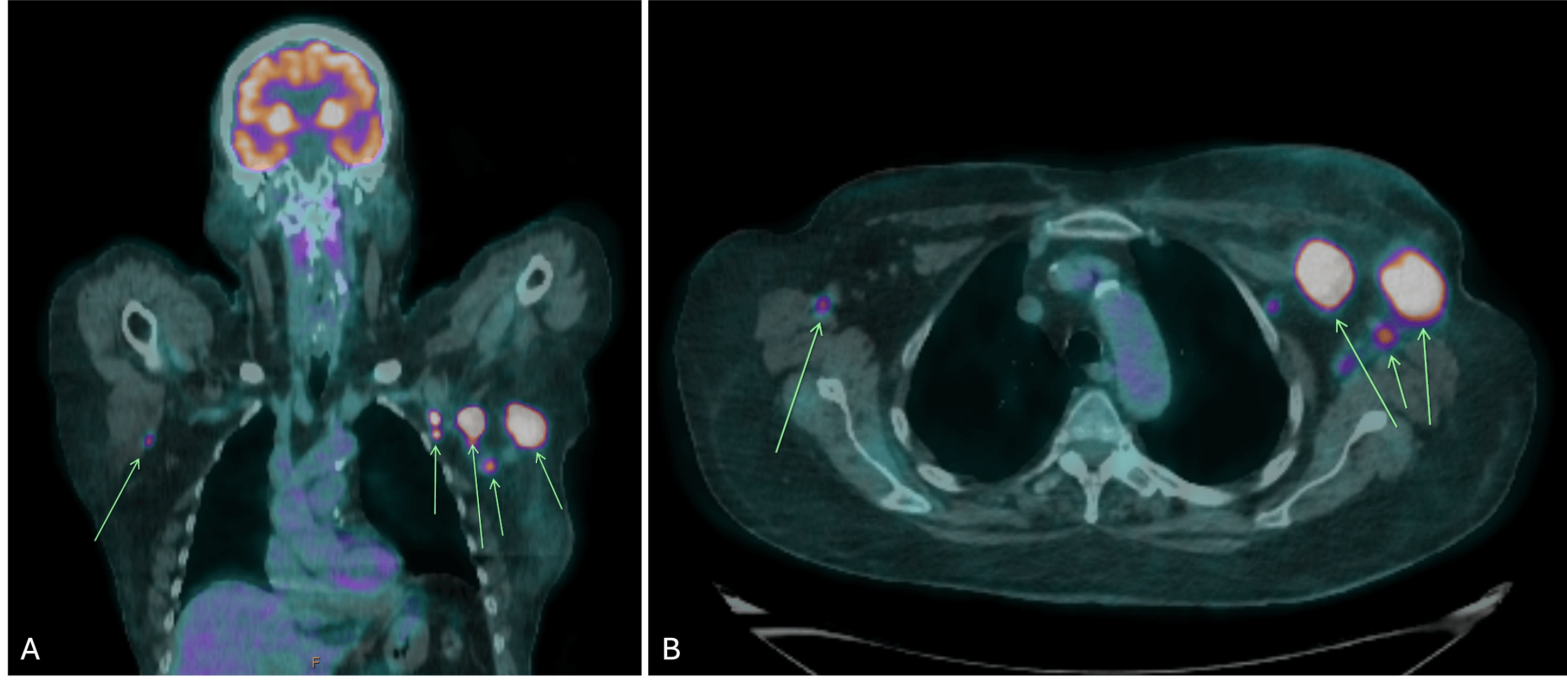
(A) Coronal view and (B) Axial view PET scan demonstrating intense abnormal FDG uptake, consistent with metastatic left axillary lymphadenopathy, with a focus of FDG avidity in the right axilla, suspicious for contralateral axillary lymph node metastasis (as indicated by arrows). PET, positron emission tomography; FDG, fluorodeoxyglucose
